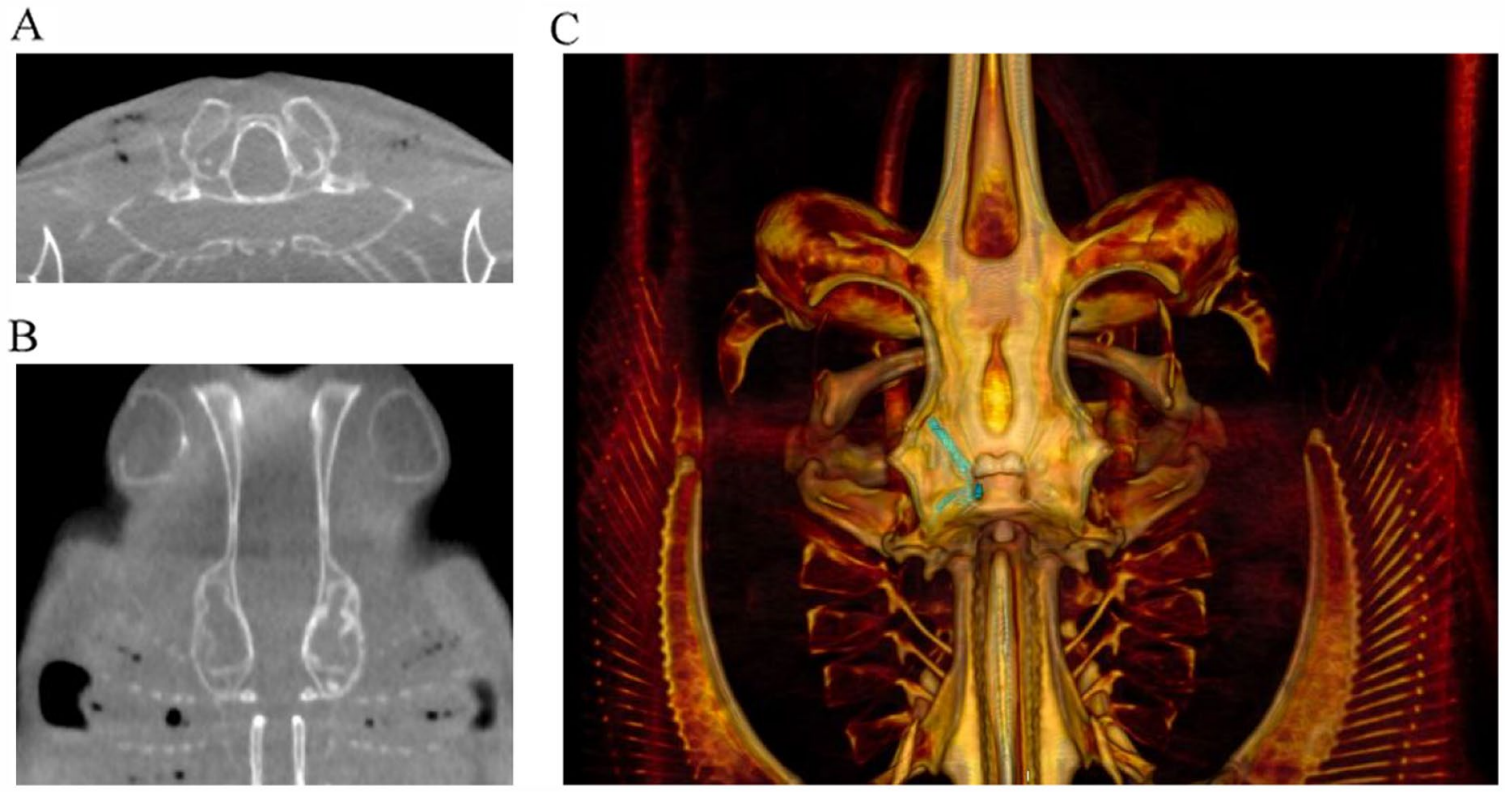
Examples of cone beam CT (CBCT) images of right-sided otitis media in a bowmouth guitarfish (*Rhina ancylostoma*). (A) Transverse plane and (B) dorsal plane image demonstrating the expansile appearance and thinning of the mineral margins of the otic capsule. (C) Volume rendered image of the same individual viewed from dorsal with the endolymphatic duct segmented in green.
